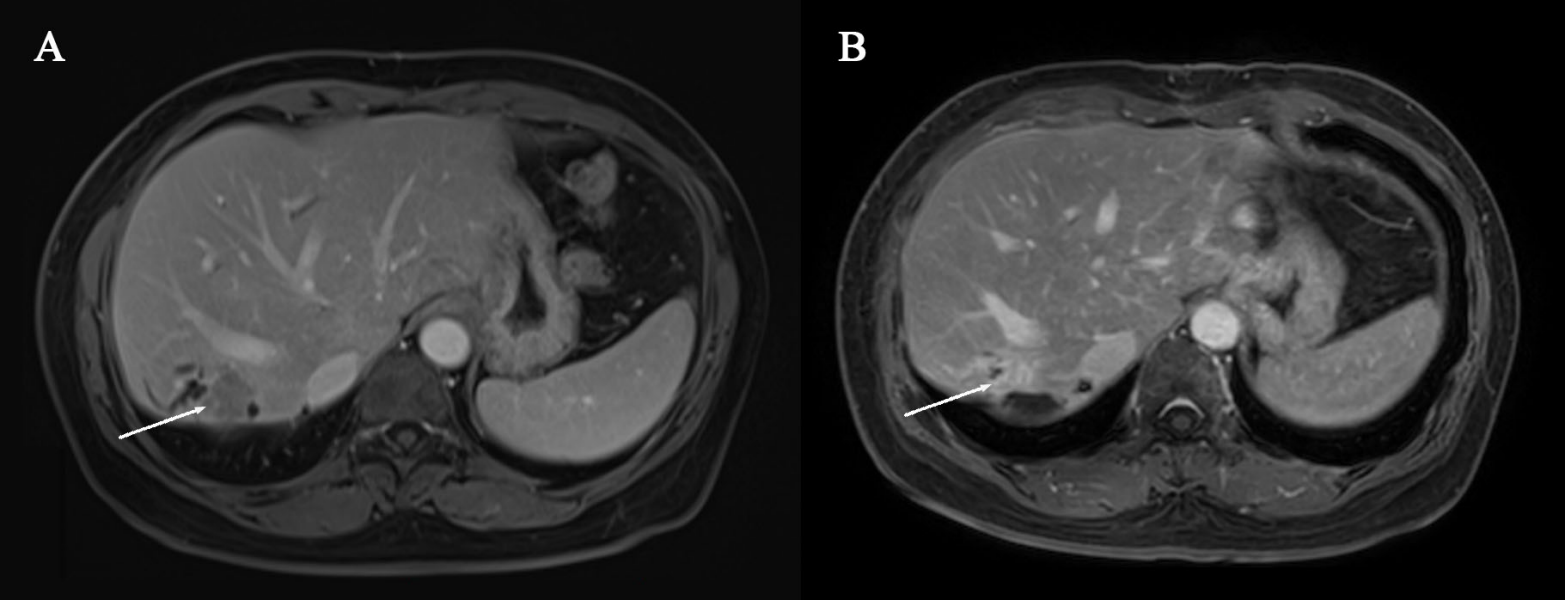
(A) Pre-systemic therapy enhanced-contrast MRI image during venous phase (B) Post-systemic therapy enhanced-contrast MRI image during venous phase. Arrow indicates tumor in (A, B).
